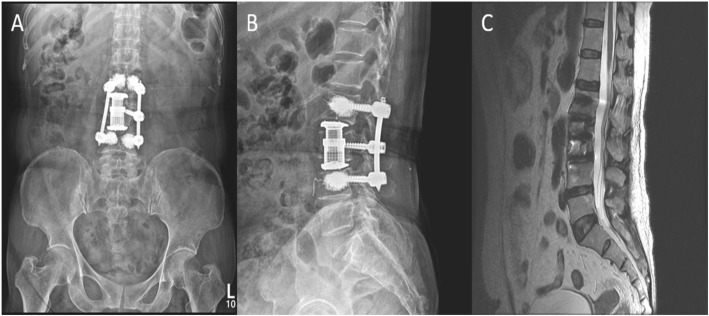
Post-operative 6 months radiograph: a AP view, b Lateral view, c 6 months follow-up MRI showing complete excision of lesion and complete resolution of osteolytic signal
